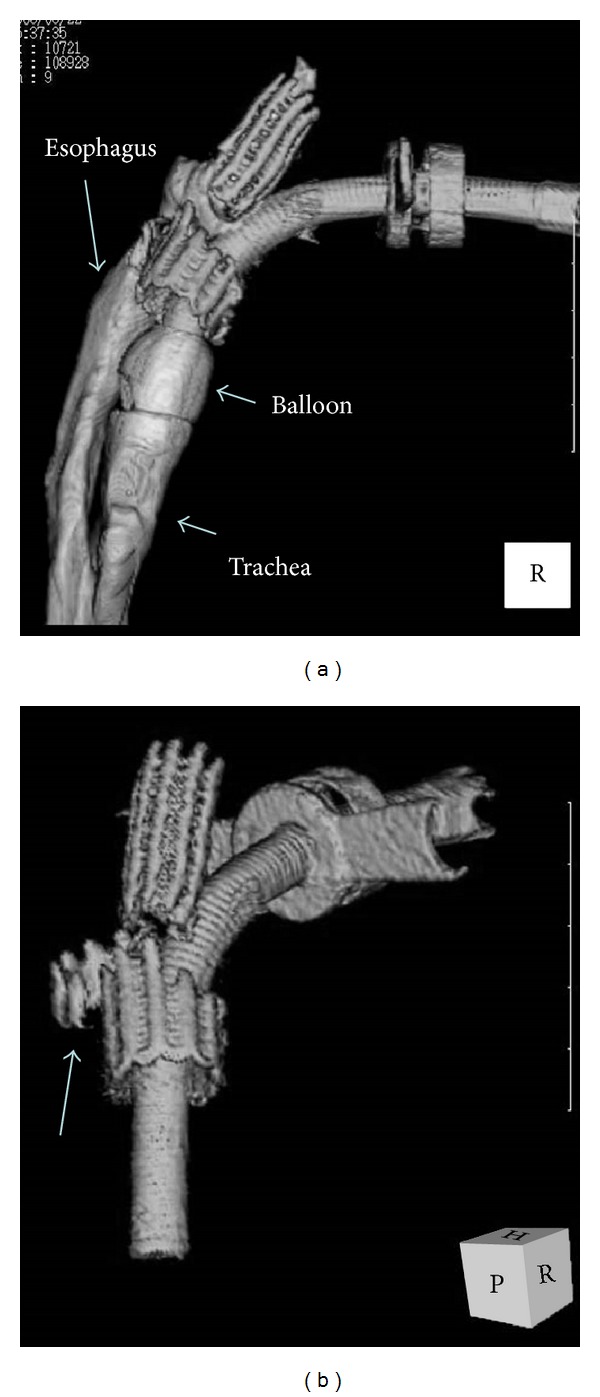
Reconstruction pictures on computed tomography. (a) The spatial relationship of the trachea, esophagus, stent, and tracheal tube was shown on CT. (b) The destroyed stent with a section existing outside of the trachea was also shown on CT (arrow).
